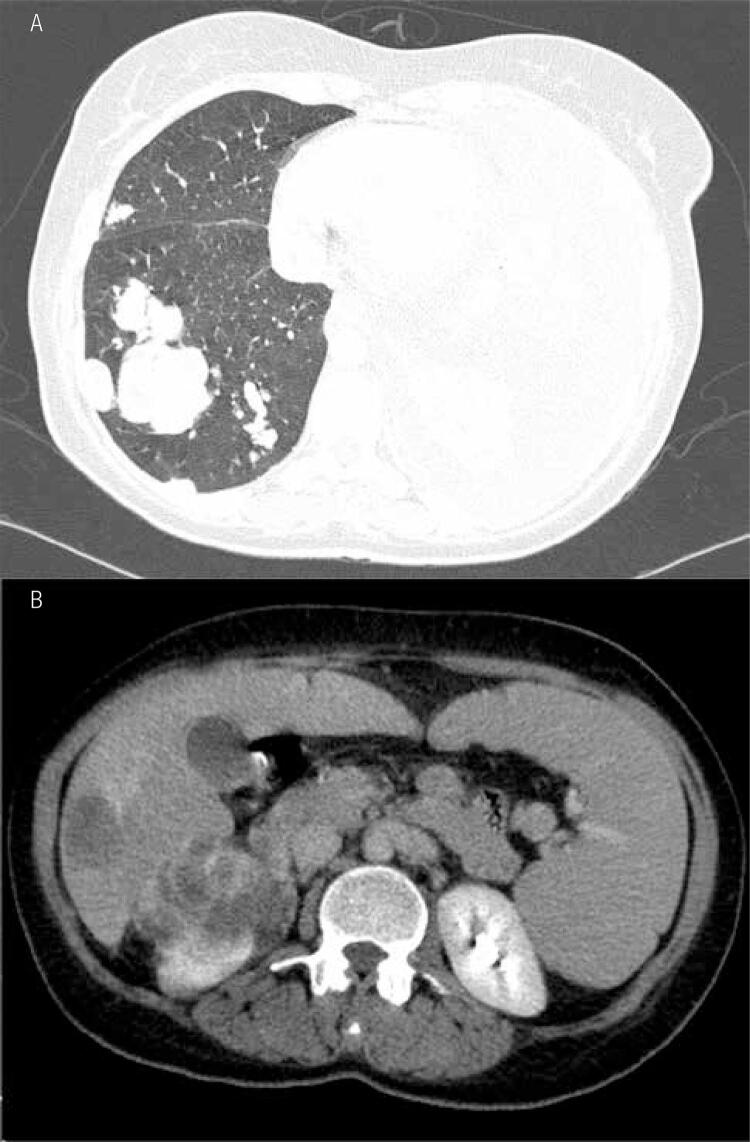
Image of chest CT from a female patient with a pT1a follicular carcinoma of 5 mm with pulmonary disease that died from obstruction of the left bronchus (A) by a metastasis six years after initial diagnosis. The patient presented also liver and kidney metastasis (B).
